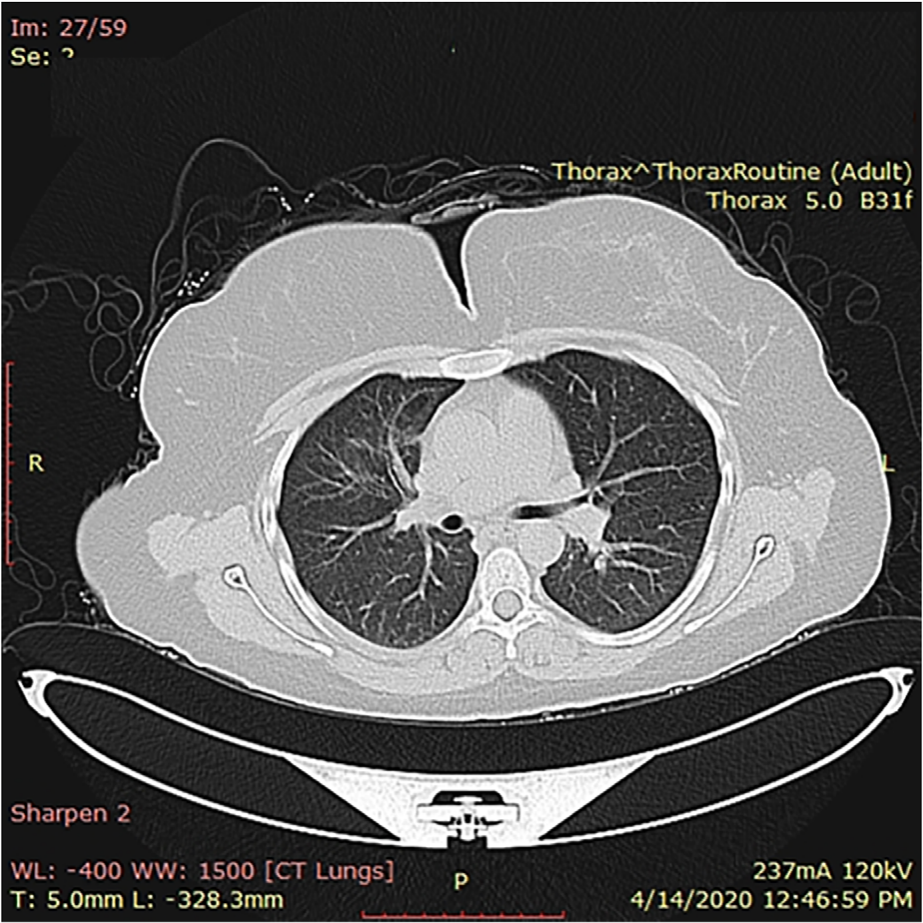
Chest computed tomography (CT) scan post treatment shows that the segmental consolidation, irregular necrosis, multiple nodules, and ground‐glass opacities in both lungs were disappeared.
